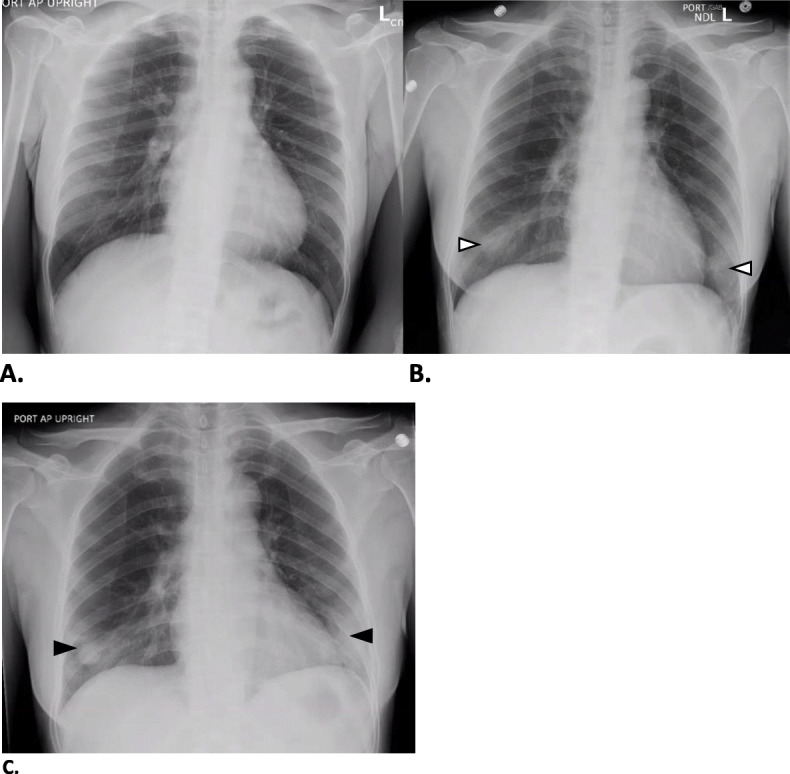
Chest X-ray from (a) hospital day 1, (b) day 3, and (c) day 6 showing serial worsening bibasilar infiltrates, White arrows: developing infiltrates, and Black arrows: worsening infiltrates
